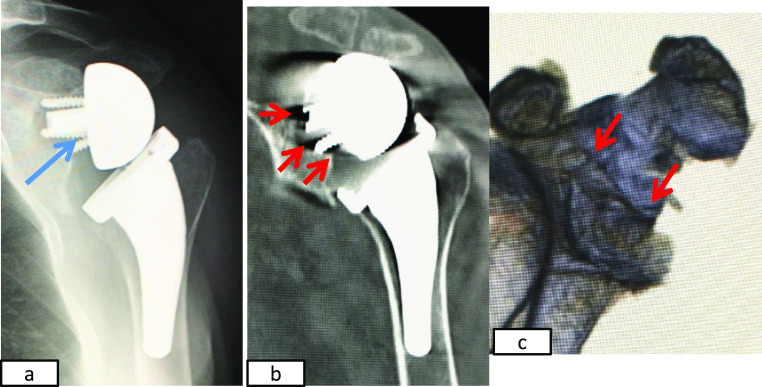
Images from 5 weeks postoperatively. a) Plain radiography shows that the baseplate is displaced superiorly (blue
